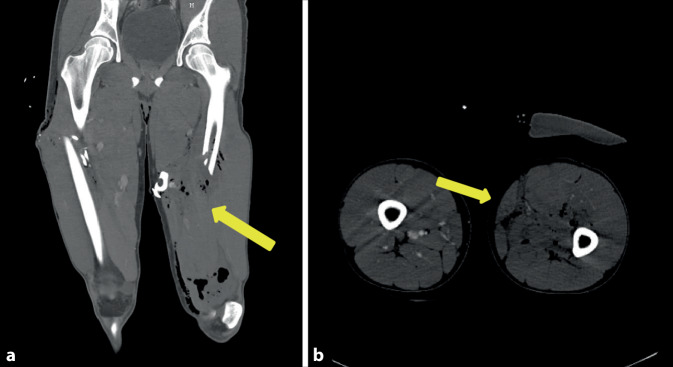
Fall 5: Traumatische Verletzung der A. und V. femoralis superficialis bei drittgradig offener Femurfraktur links. a CT-Angiographie in koronarer Schichtung mit Darstellung der Oberschenkelfraktur und Lufteinschlüssen (*gelber Pfeil*), b CT-Angiographie in axialer Schichtung mit fehlender Kontrastmittelfüllung der V. und A. femoralis superficialis links (*gelber Pfeil*)
